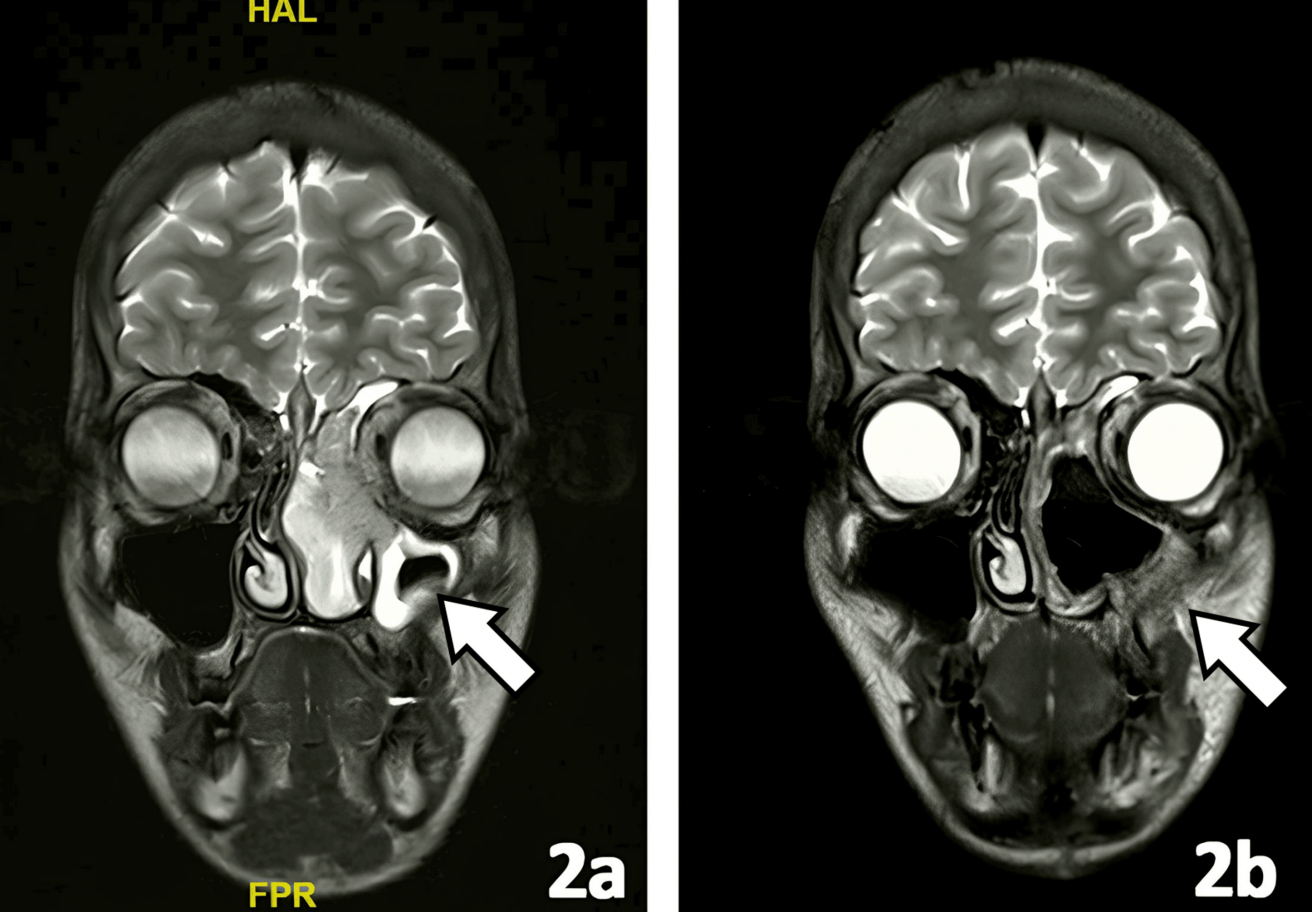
MRI coronal section images (a, b) MRI images of a patient before and after curative-intent treatment (site of primary lesion shown with an arrow mark)
